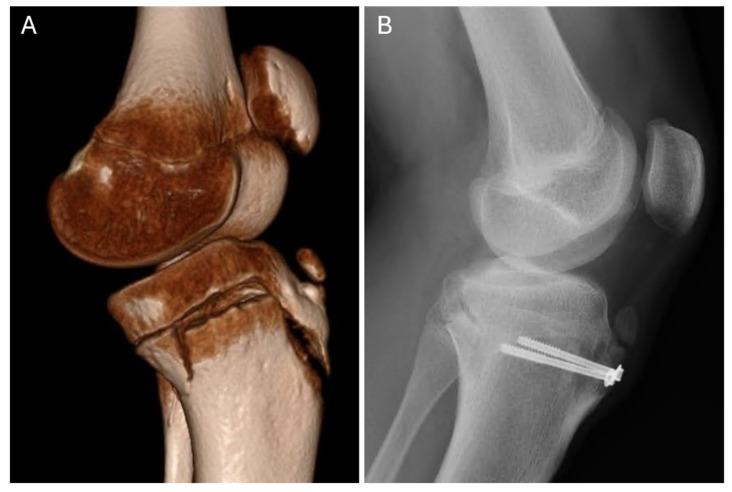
Three-dimensional computed tomography and postoperative radiographs demonstrating an Ogden type IVb tibial tuberosity fracture with residual changes of Osgood–Schlatter disease. (A) A 3D-CT reconstruction of a type IVb tibial tuberosity fracture with residual Osgood–Schlatter disease. (B) Postoperative radiograph taken 10 weeks after osteosynthesis with two cancellous screws and washers, showing a healed fracture.
